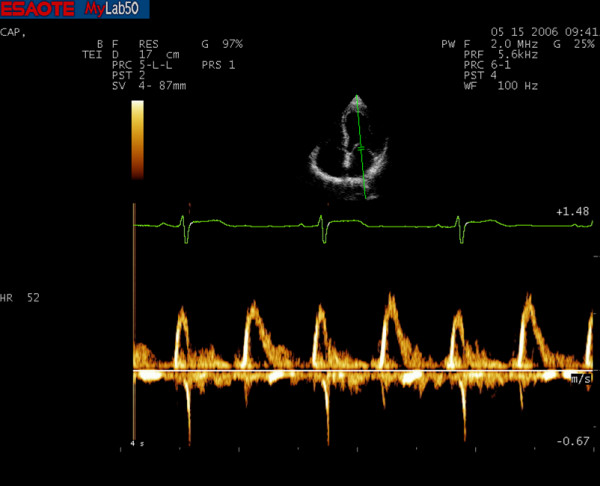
Conventional PW Doppler spectrum of a master athlete's heart.
